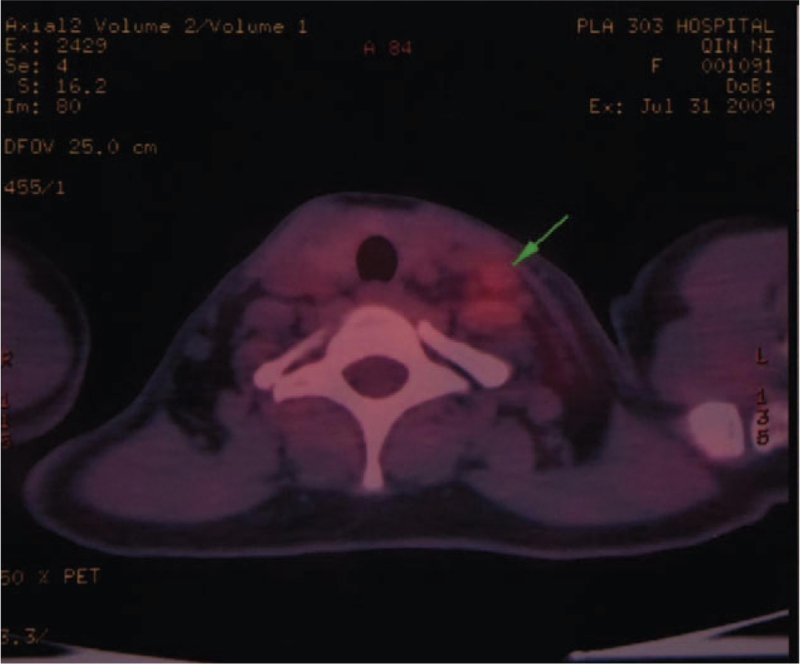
The whole body F18-FDG PET/CT tomography scan (July 31, 2009) showing multiple enlarged lymph nodes on left supraclavicular area. F18-FDG-PET/CT = Fluorine 18 fluorodeoxyglucose- Positron emission tomography/computed tomography.
